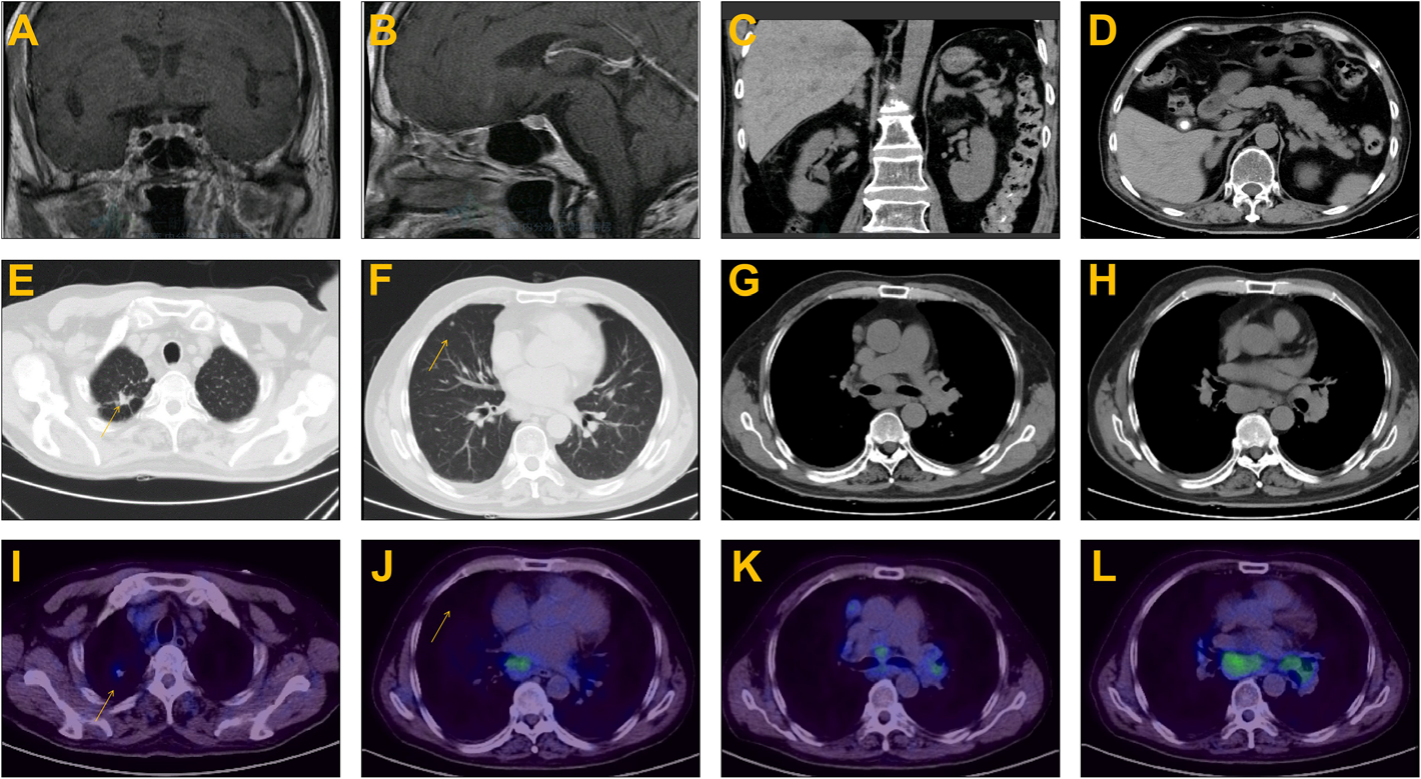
Imaging examinations. Pituitary MRI revealed no abnormalities (a and b). Abdominal CT showed diffuse enlargement of the bilateral adrenal glands (c and d). Chest CT indicated two nodules in the superior (e) and middle lobes of the right lung (f) and mediastinal lymphadenopathy (g and h). ^68^Ga PET/CT demonstrated high-density opacity in the superior lobe of the right lung with a slight abnormally increased uptake of ^68^Ga-DOTA-NOC (i). High-density nodules in the middle lobe of the right lung did not show abnormal uptake of ^68^Ga-DOTA-NOC (j, arrow). Multiple lymph nodes in the mediastinum and bilateral hili of the lung partially fused into a mass with abnormally increased uptake of ^68^Ga-DOTA-NOC (k and l)
